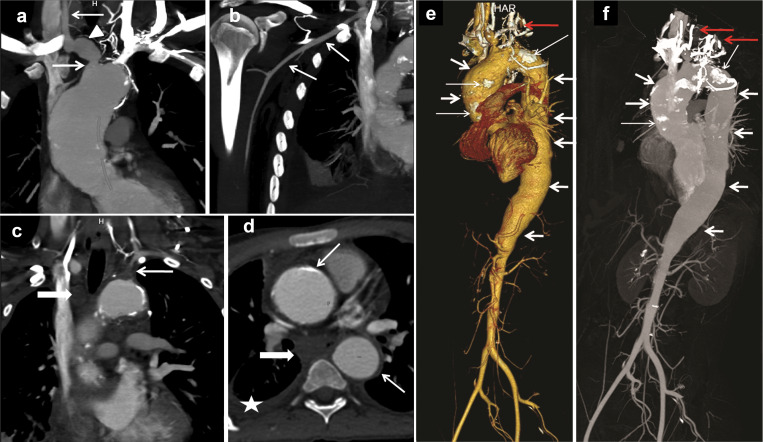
Panel of CT angiography images showing aneurysmal dilataion of aorta and its branches with development of collaterals and mediatstinal lymphadenopathy in patient 1. (a–d) Panel of CT angiogram images of patient 1: reconstructed coronal images (a and b) show focal stenosis at the origin of the right brachiocephalic trunk (solid white arrow below the arrowhead) with poststenotic dilation (arrowhead). Right common carotid (solid white arrow above the arrowhead in a) and subclavian (arrows in b) are normal. Reconstructed coronal image (c) shows nonopacified (occluded) proximal left subclavian artery until the origin of the vertebral artery (arrow). Note the left common carotid artery is also not opacified. Conglomerate necrotic lymph nodes are seen in right paratracheal location (thick arrow in c). Axial image (d) shows circumferential mural thickening of the ascending and descending thoracic aorta with mural calcifications (thin arrows). Note necrotic subcarinal lymphadenopathy (thick arrow) and right pleural effusion (asterisk). (e and f) CT angiogram volume rendered (e) and maximum-intensity projection (f) images show aneurysmal dilatation of the aorta extending from the aortic root up to the suprarenal part of the abdominal aorta (thick white arrows). Note multiple mural calcifications (thin white arrows) and multiple collaterals in the neck (red arrows). Visceral arteries including renal and iliac arteries are normal.
